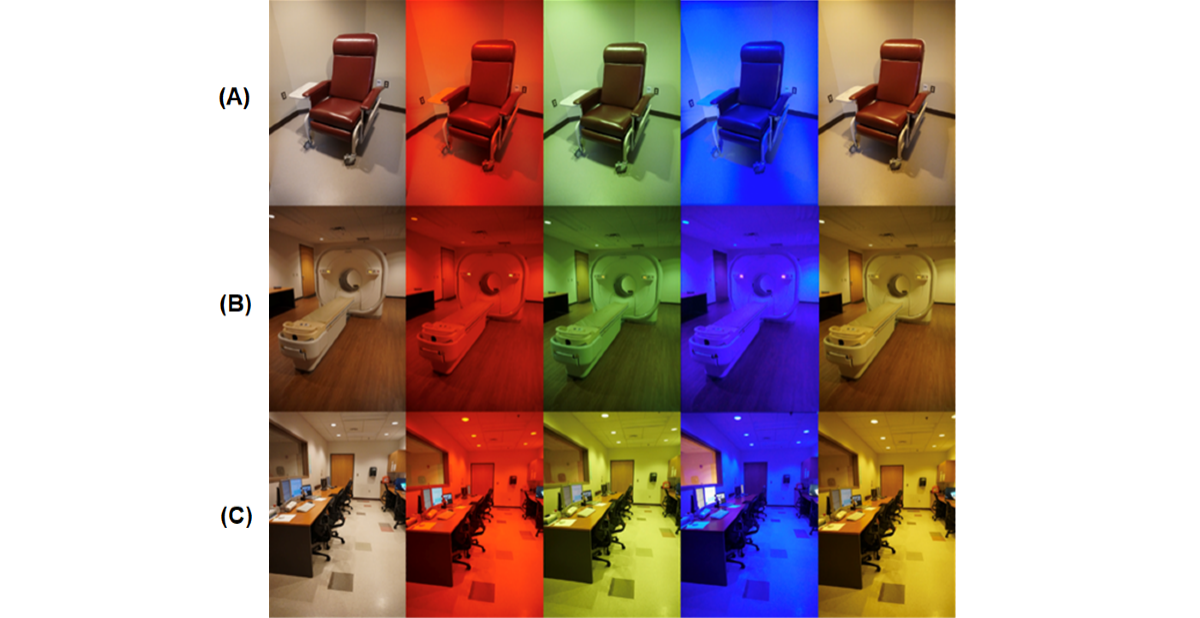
Different color settings in three rooms within an imaging environment. (A) Patient injection/preparation room; (B) scan room of the digital positron-emission tomography/computed tomography (PET/CT) system; and (C) PET/CT control room. In each of these rooms, different color settings are demonstrated as follows: standard bright white light, red light, green light, blue light, and yellow light.
